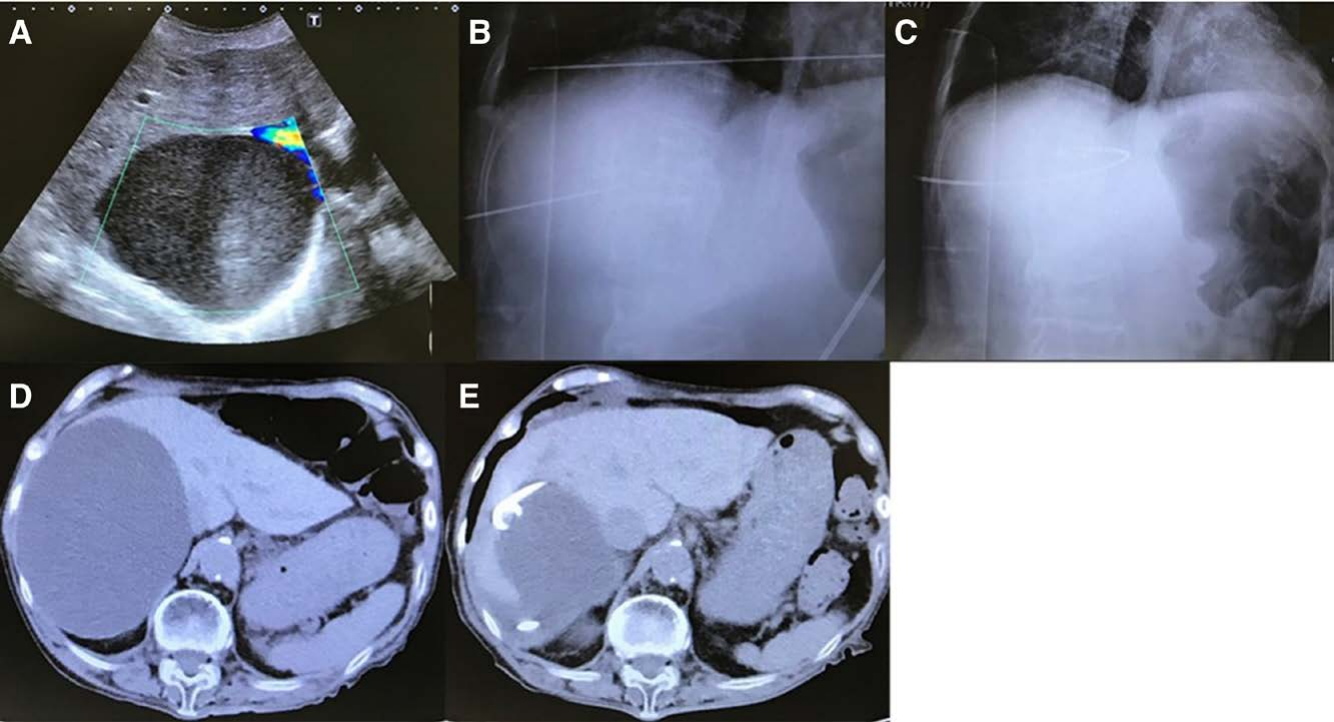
PCD with minocycline sclerotherapy for treating symptomatic SHC. (A) Ultrasound indicates a large cyst in right lobe of the liver. (B) An 18-gauge needle is advanced into the cavity. (C) A 7-Fr pigtail long catheter is placed. (D) CT scan reveals a large cyst in right lobe of the liver. (E) CT scan confirms that the drainage tube is placed in the cavity of the hepatic cyst. CT = computed tomography, PCD = percutaneous catheter drainage, SHC = simple hepatic cysts.
